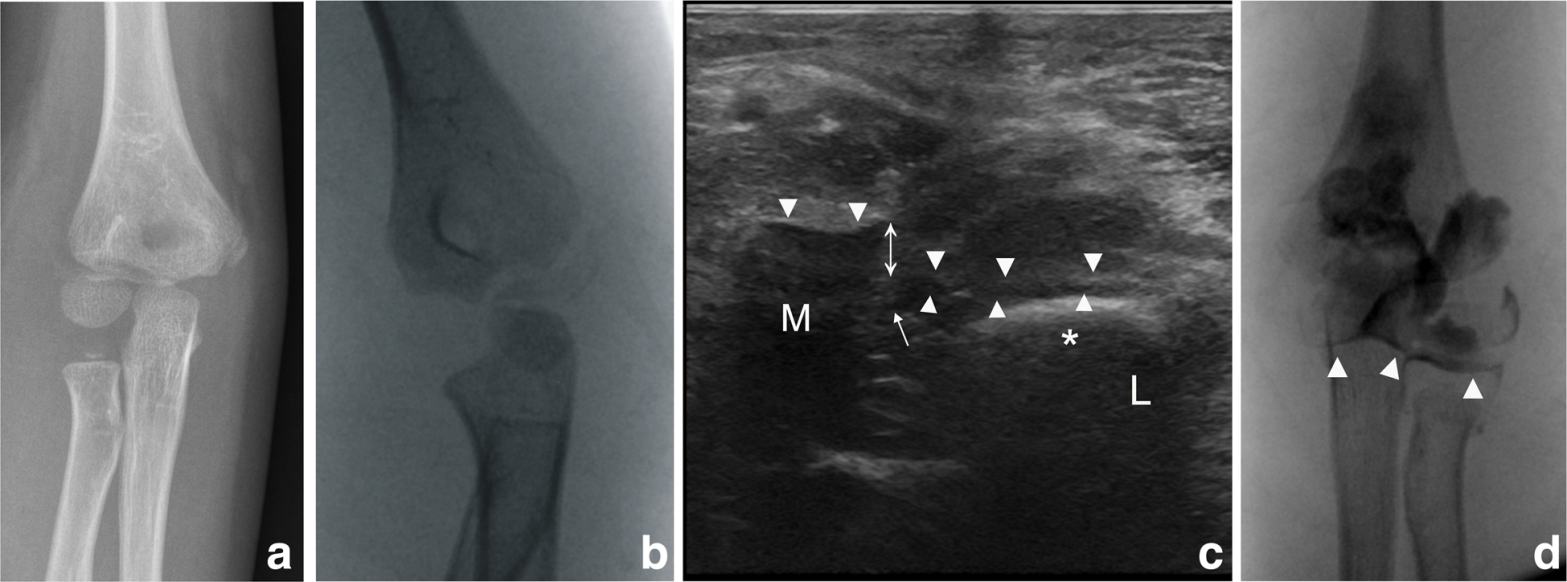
Patient case 4. a, b Radiographs in the anteroposterior and internal oblique views. c The transverse ultrasound image showed the disrupted cartilage hinge and the stair sign in a large cartilage gap. d Radiographs of anteroposterior views of an arthrogram. The dye continued along the fracture line and passes through the articular surface, thus indicating an incongruity of the articular surface. Arrow, disrupted cartilage hinge; arrowhead, cartilage hinge; Asterisk, epiphyseal core of ossification of the capitellum; M, medial; L, lateral
